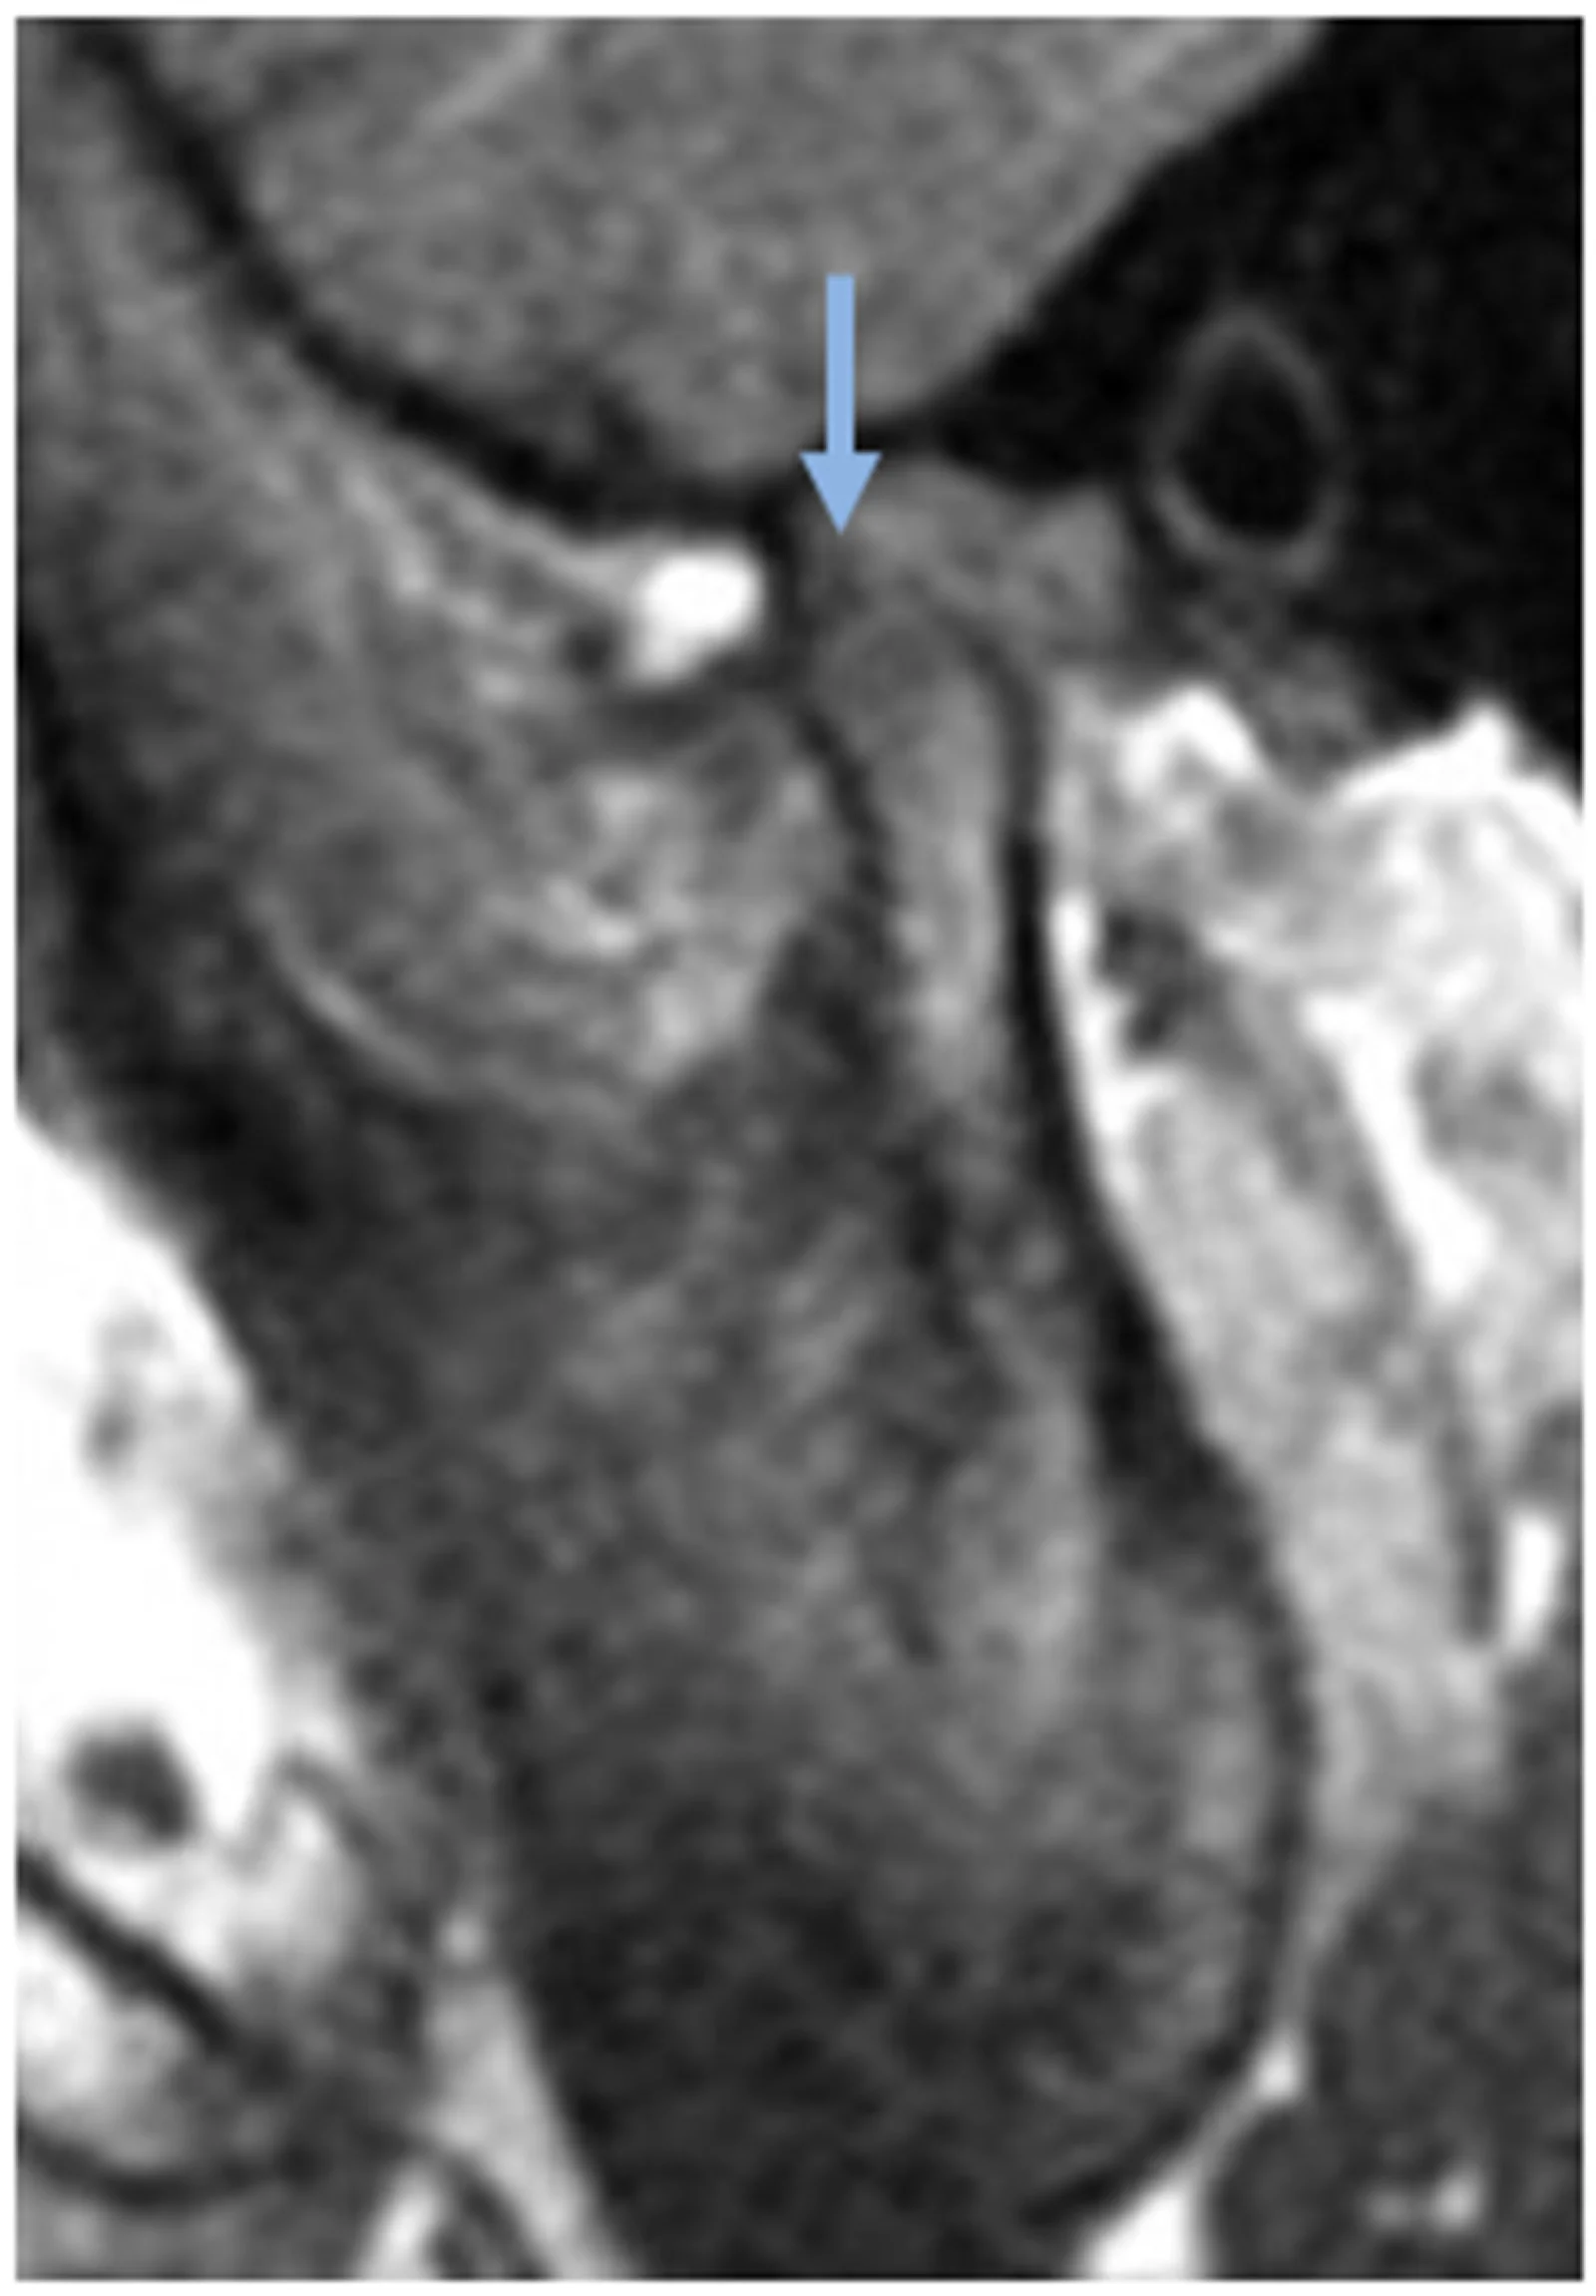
Magnetic resonance imaging of the viscerocranium in the sagittal plane, demonstrating the left temporomandibular joint of a female patient diagnosed with Juvenile Idiopathic Arthritis at the age of 12 years. The left temporomandibular joint (blue arrow) demonstrates mild condylar bone marrow edema, with imaging features suggestive of early erosive osseous changes. Anterior displacement of the articular disc without reduction during mouth opening is also observed, consistent with internal derangement.
